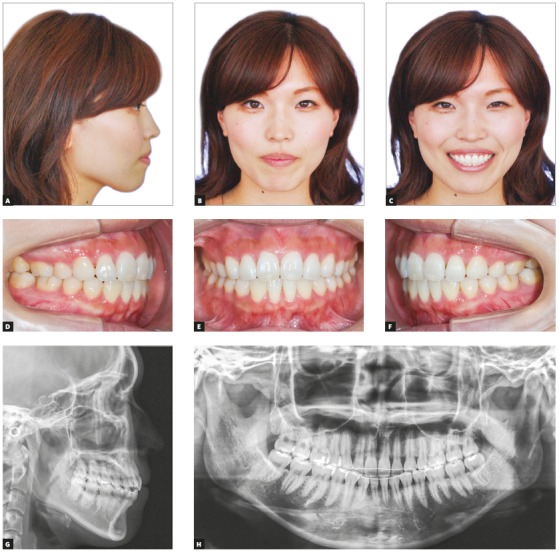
Post-retention records (at 26 years and 7 months of age): A-F) extraoral and intraoral photographs, G) lateral cephalometric radiograph, H) panoramic radiograph.
